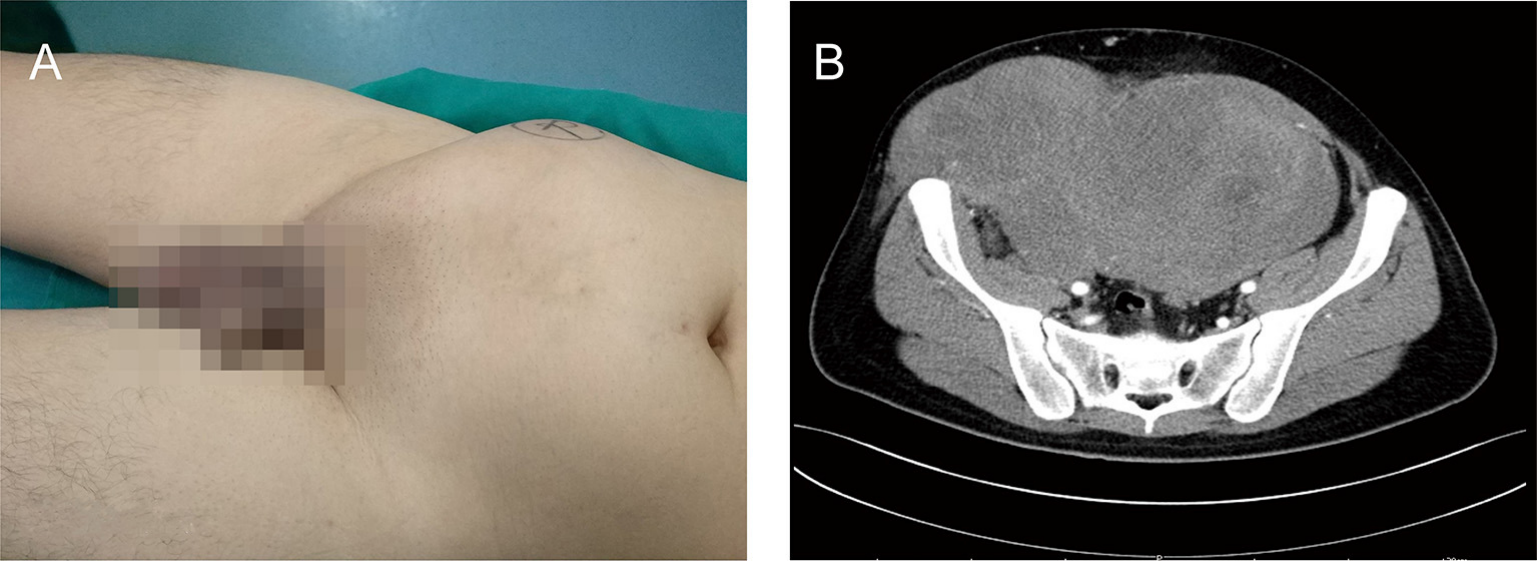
A clinical presentation of a giant right abdominal mass before surgical treatment. (A) The giant mass of right abdomen was visibly prominent. (B) The preoperative contrast-enhanced CT showed a large, inhomogeneous mass in the right abdominal wall that invaded right testis, spermatic cord, and right abdominal wall muscles, and demonstrated mild enhancement.
